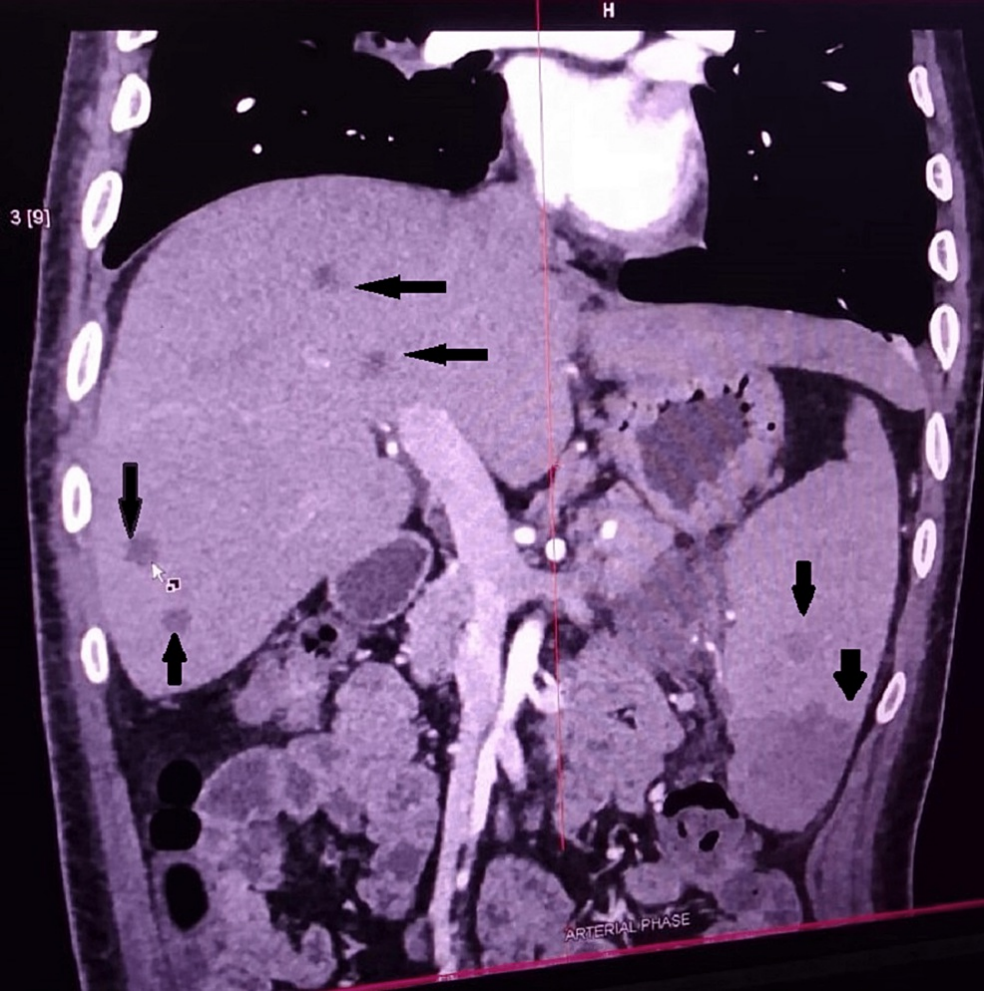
CT abdomen image showing multiple liver abscesses, splenic abscesses, and splenic infarcts in the coronal section
